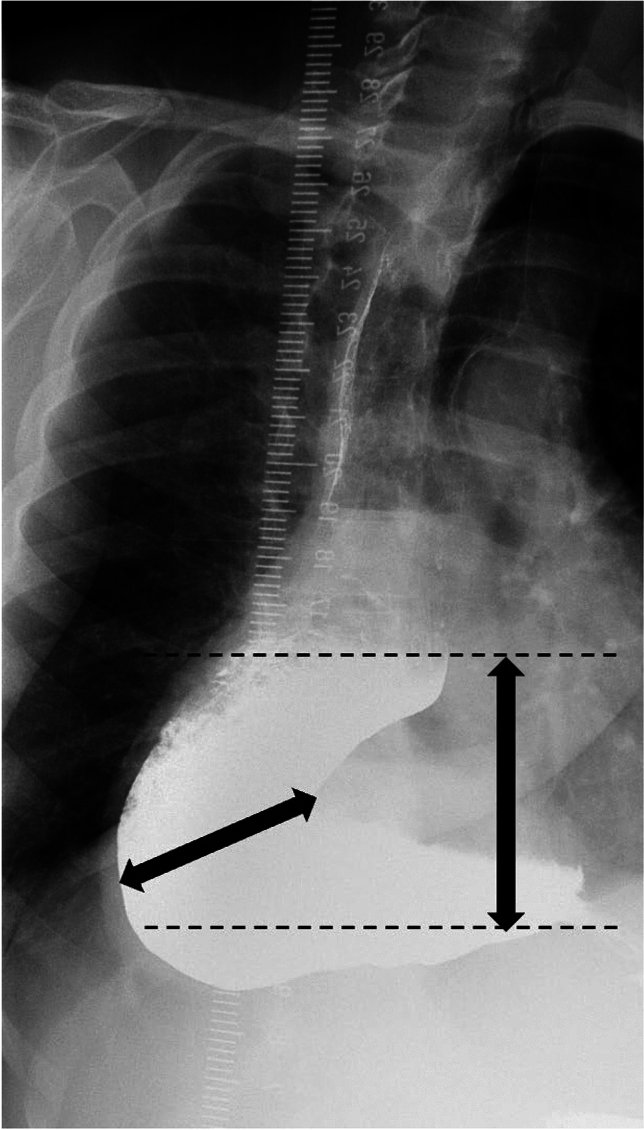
An example of column height and width measurements. LPO radiograph 5 min after ingestion of barium in type 1 achalasia in a patient with a sigmoid esophagus
